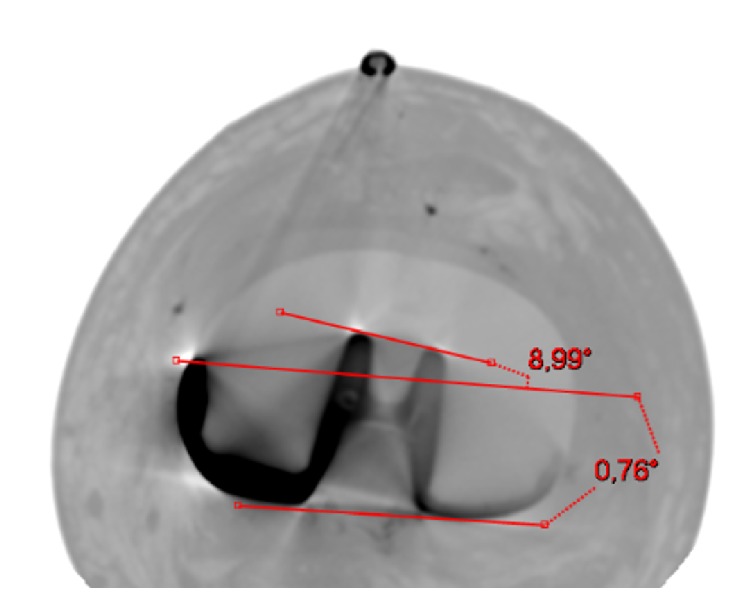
Left knee. Tibial component (Persona, The Personalized Knee System, Zimmer, Warsaw, USA) computed tomography (CT) axial view. Two angles were measured: (1) PCA (posterior condyles angle): angle between the projected surgical transepicondylar axis (sTEA) and the line of the posterior condyles (0.76°); (2) the angle between the projected sTEA and the mediolateral axis of the tibial component (8.99°). The mediolateral axis of the tibial baseplate has been identified through its “dwell points.” This tibial baseplate is externally rotated by 8.99° with respect to the projected femoral transepicondylar axis (sTEA).
